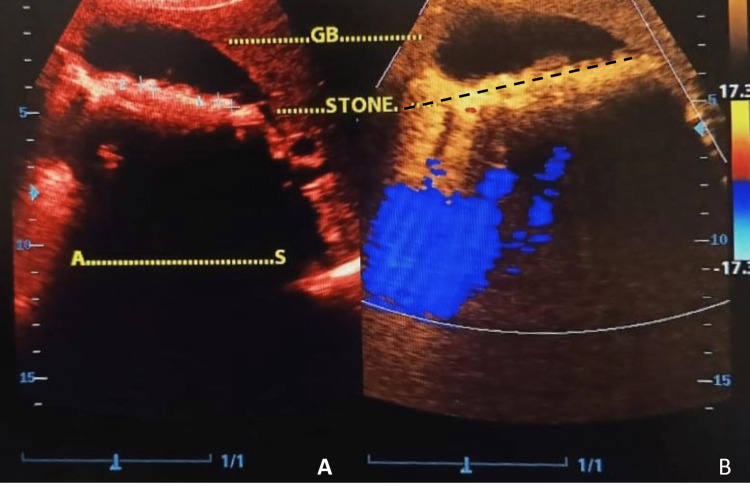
(A and B) Abdominal ultrasonography image (B-mode) showing distended gall bladder with stones, sludge, thickened gall bladder walls, and signs of cholecystitis GB: Gall bladder.
